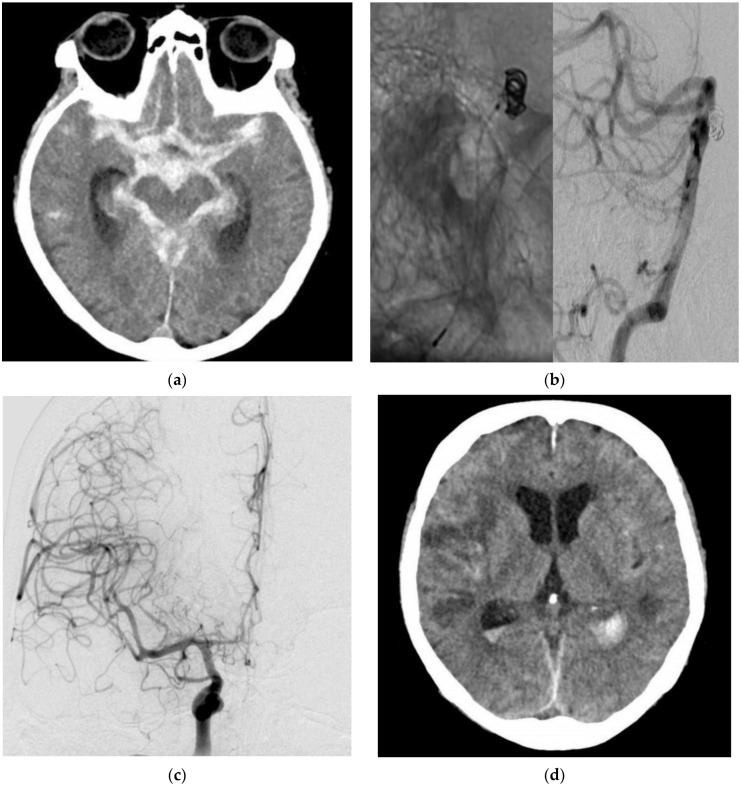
Diagnostic imaging and EVT in a 70-year-old female patient with an aSAH due to a ruptured basilar artery aneurysm. CT (a) showed a massive aSAH. DSA (b) with contrast-medium injection of the left vertebral artery (VA; lateral projection, asterisk) demonstrated the EVT with the coil mass inside the aneurysm. DSA (c) with contrast-medium injection of the right internal carotid artery (ICA; posterior–anterior projection) revealed massive vasospasm. Post-interventional CT (d) showed ischemic infarctions despite EVT.
